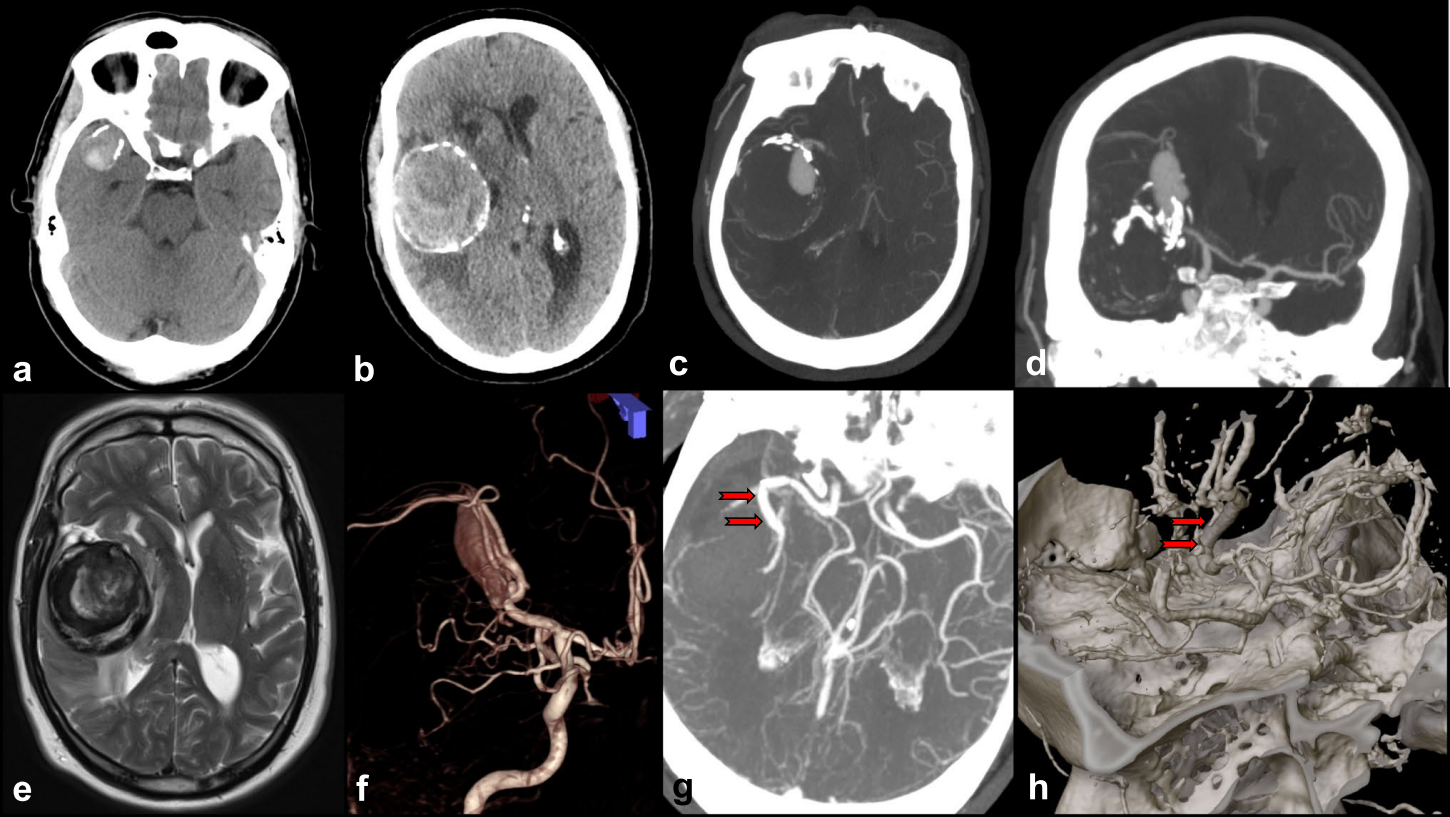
A 65-year-old man, hypertensive, smoker, and with previous TIAs, was diagnosed with a large right MCA aneurysm 13 years prior that was managed conservatively (a). He presented with progressive left hemiparesis, impaired conscious level. b CT head showed a heavily calcified giant right MCA aneurysm with surrounding edema. c, d CT angiogram (axial and coronal views) showed patent aneurysm remnant, heavy calcification at the aneurysm neck, and thick layers of intraaneurysmal thrombus. e MRI brain, T2-weighted, axial view showed onion-ring appearance of multilayered intraaneurysmal thrombus. f 3D reconstructed angiogram showed single MCA branch arising from the aneurysm neck. g He underwent aneurysmectomy with in situ MCA end-to-end anastomosis using interposition saphenous vein graft (IC-IC bypass) and made good recovery. At 6 months follow-up, he was independently mobile, with resolution of hemiparesis. g, h CT angiogram confirmed patent in situ bypass graft (red arrows)
